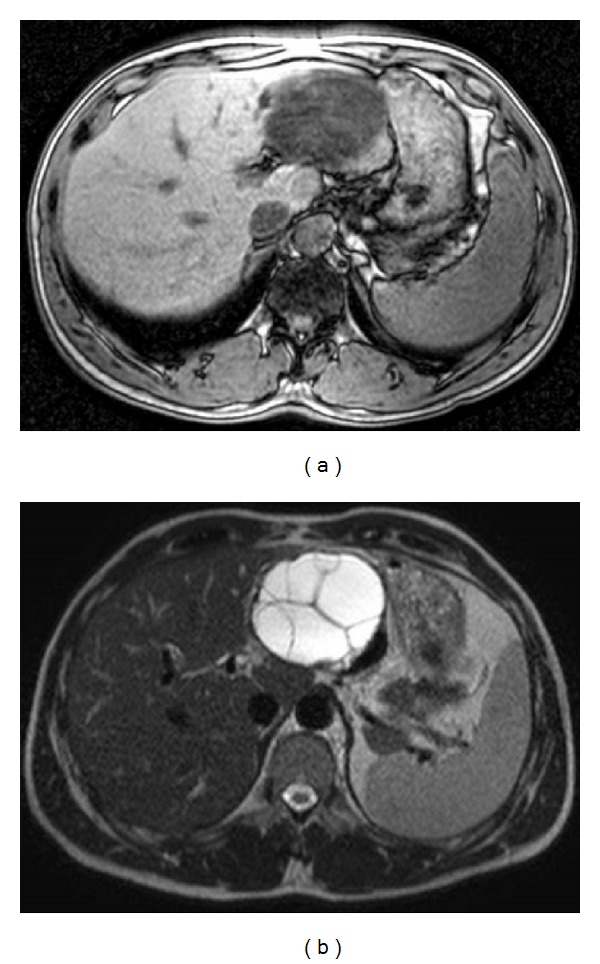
Magnetic resonance imaging of this tumor revealed low contrast intensity in the T1 weighted image (a) and high intensity in the T2 weighted image (b).
